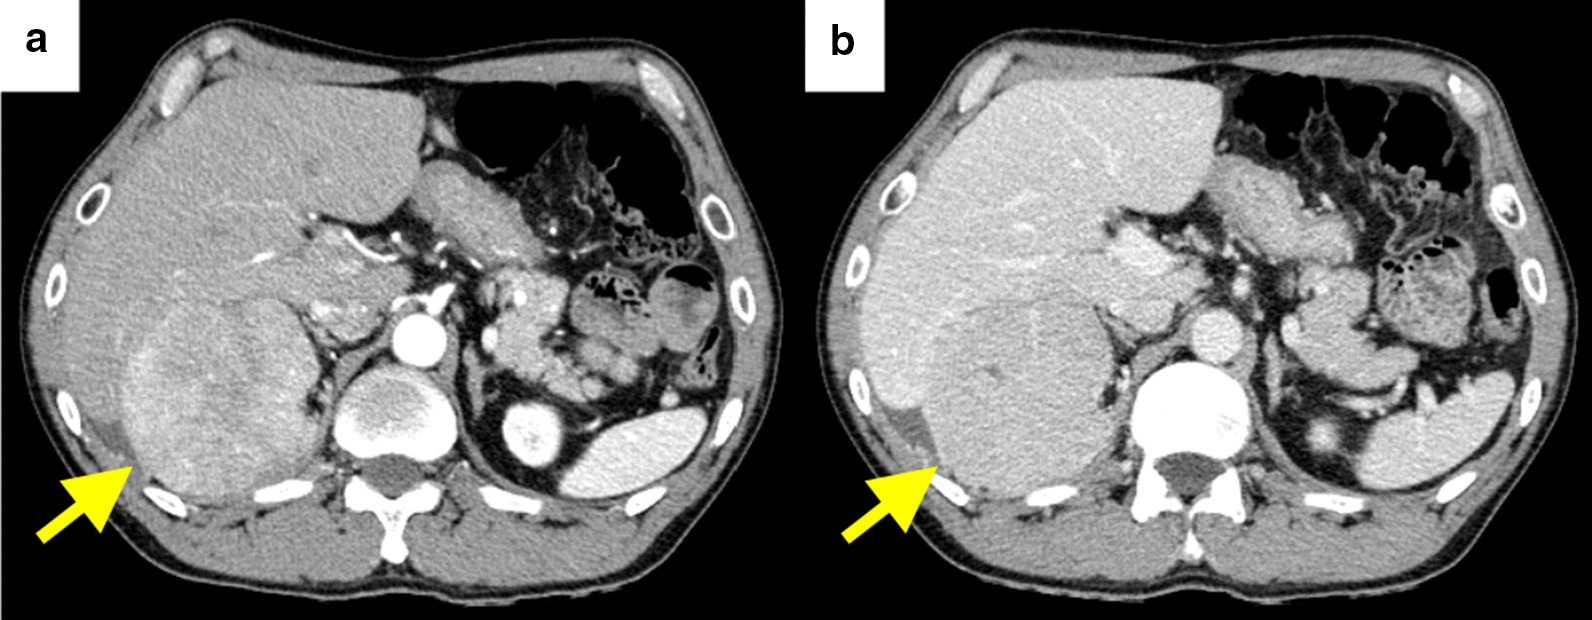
Contrast-enhanced abdominal computed tomography images showing a 10 cm diameter tumor protruding from segment 6 into the retroperitoneum. a Arterial phase. b Portal phase
